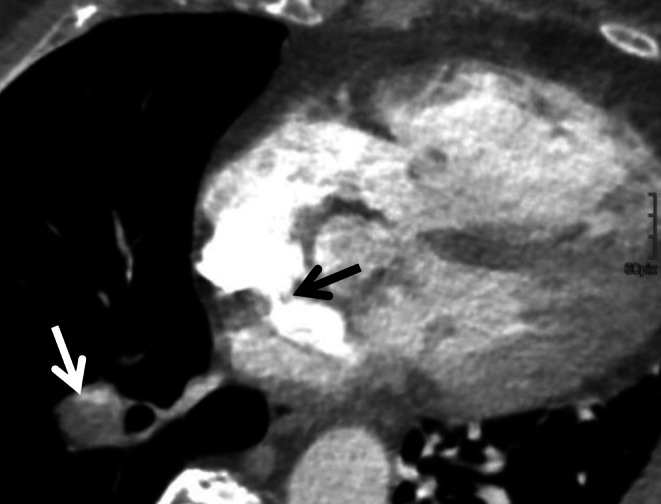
Image section of computed tomography in axial view of the heart. In this patient, a fulminant pulmonary embolism (*white arrow*) was observed in addition to the cerebral large-vessel occlusion. In cardiac imaging as part of the CS-CT protocol, a contrast jet in atrial septal defect with right-left shunt could be seen (*black arrow*), presumably as a cardiac cause of stroke due to additional paradoxical embolism. A TEE was not possible due to the poor general condition of the patient
